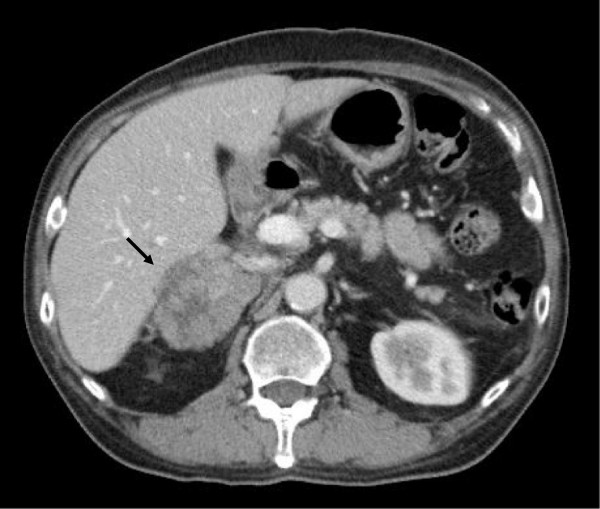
Abdomen contrast computed tomography (CT) showed a 7.8 × 4.8 cm heterogenous lobulated enhancing mass lesion (arrow) at right adrenal gland with suspicious local invasion to the posterior segment of liver.
